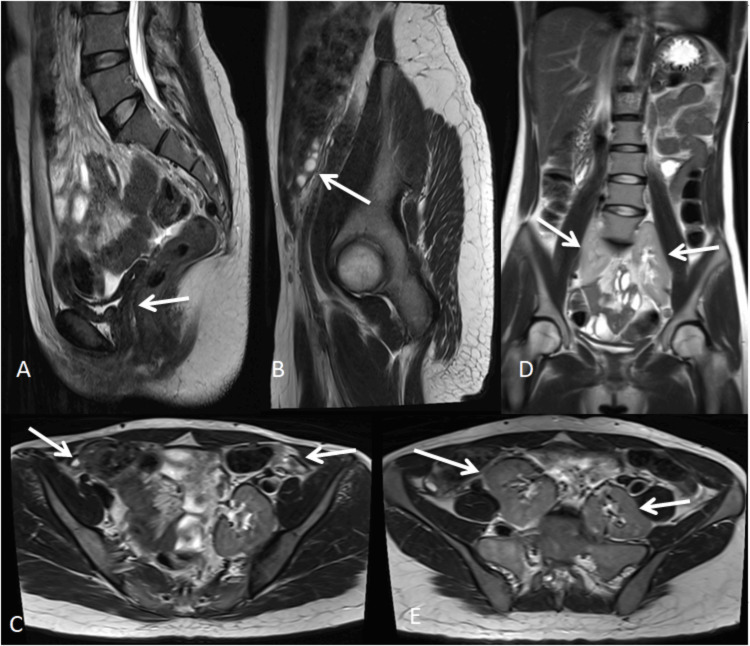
Mayer-Rokitansky-Küster-Hauser syndrome type B (A) Sagittal T2-weighted image demonstrating the absence of the uterus, cervix, and upper two-thirds of the vagina (white arrow), consistent with Müllerian agenesis. (B) Sagittal T2-weighted image showing normal-appearing ovaries with follicles (white arrow). (C) Axial T2-weighted image confirming normal bilateral ovaries (white arrows). (D) Coronal T2-weighted HASTE image showing the absence of kidneys in the renal fossae (white arrows) with ectopic pelvic kidneys. (E) Axial T2-weighted image demonstrating bilateral ectopic kidneys in the presacral region with inferomedially directed hila (white arrows). HASTE: Half-Fourier-acquired single-shot turbo spin echo.
